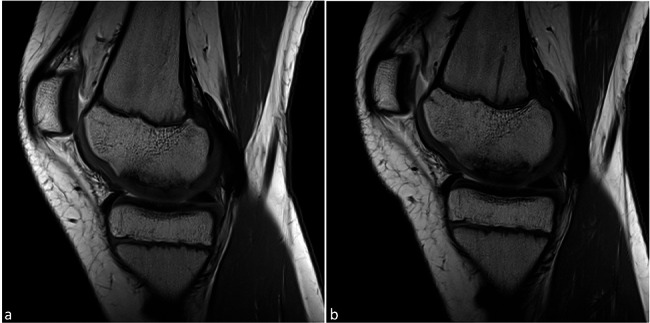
Typical presentation of T1-weighted turbo spin echo sequences (T1-TSE) follow-up MR images in the sagittal plane of stage I osteochondrosis dissecans in the right knee joint of twin 2: a stable lesion with moderate osteolysis and reactively thickened but intact cartilage cover from *(a)* the stage at the onset of symptoms and *(b)* after 5 months
